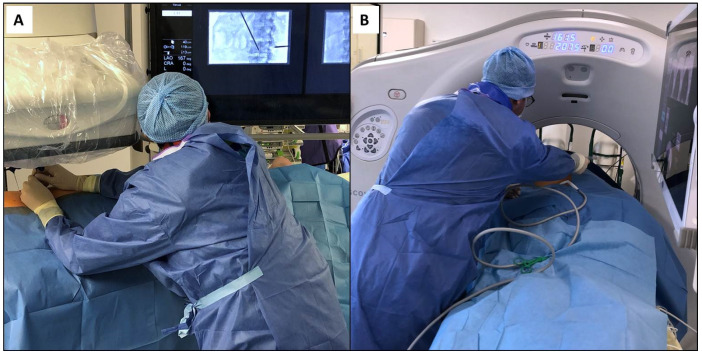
Percutaneous procedures performed under image guidance often require grip strength with instruments, bending for adequate needle positioning, and cervical rotation to visualize the screens: (A) vertebroplasty performed under cone-beam computed tomography; (B) cryoablation performed under computed tomography guidance. The resulting body contortion potentially leads to discomfort and musculoskeletal disorders.
